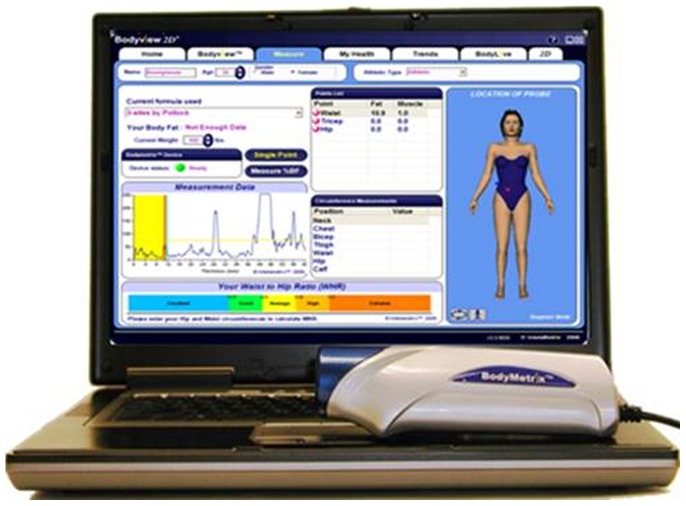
Image of the ultrasound transducer and corresponding software used for evaluation.
